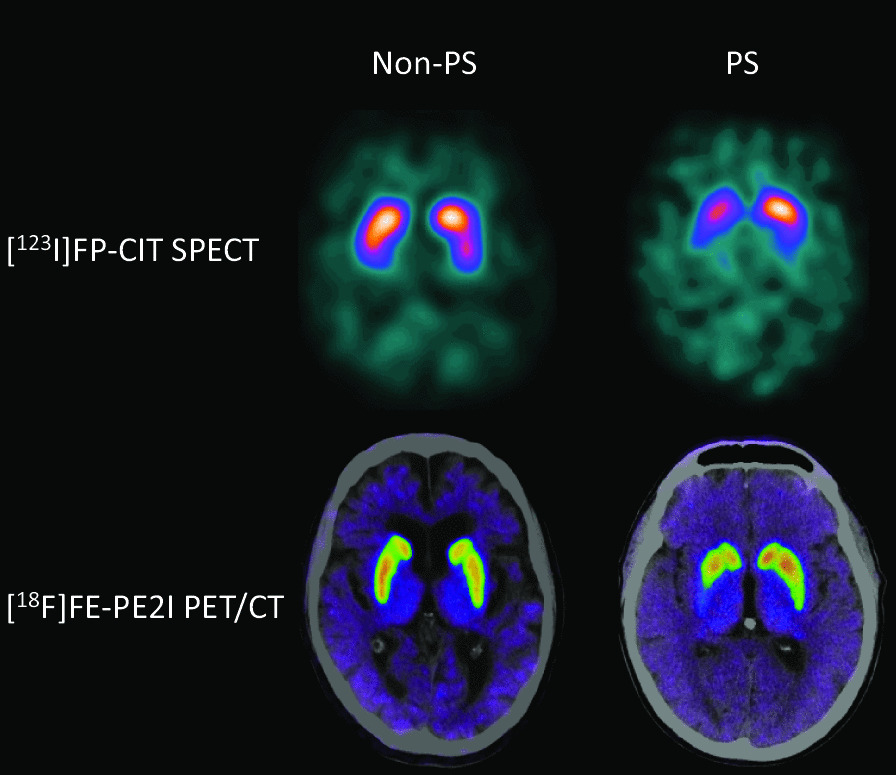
Sample images of two patients evaluated with [^123^I]FP-CIT SPECT and [^18^F]FE-PE2I PET. PS: Parkinsonian syndromes
